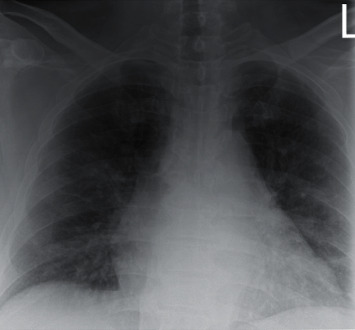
Chest X-ray showing multifocal patchy airspace disease, COVID-19.
